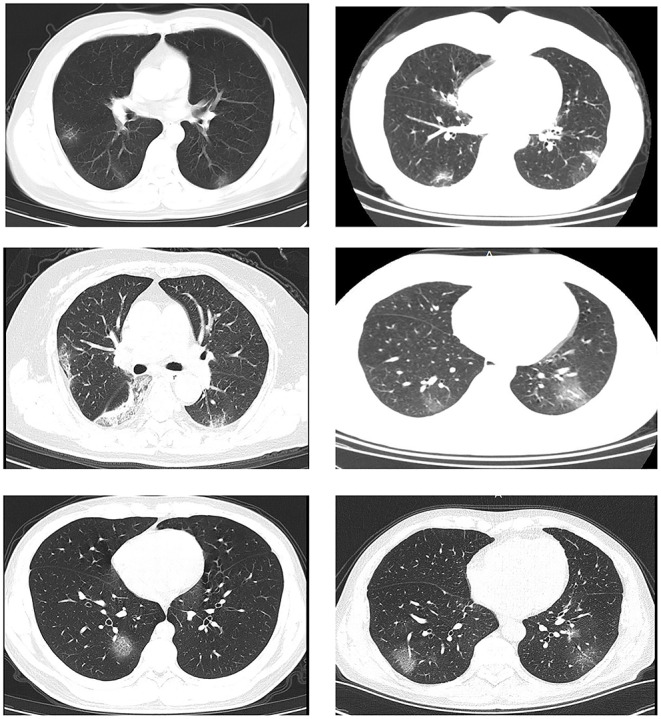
Transverse chest computed tomography images of patients with COVID-19. Transverse chest computed tomography of six patients with COVID-19 on admission showed bilateral or multiple lobular or subsegmental areas of consolidation or bilateral ground glass opacity.
